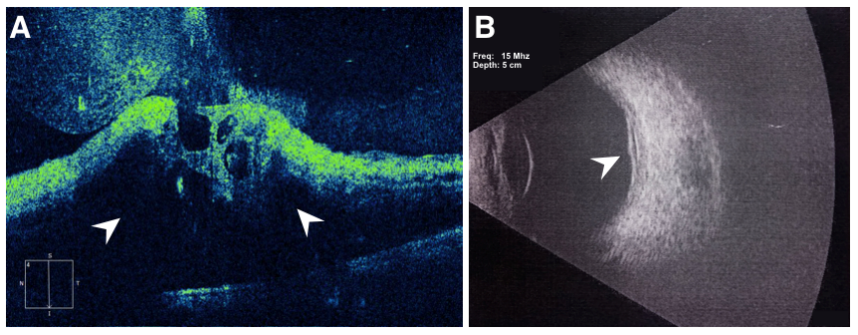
The optical coherence tomography image shows extensive macular edema seen as hyporeflective regions within the retina (arrowhead, 2A). Back shadowing due to superficial hemorrhages is also seen. Ultrasound imaging shows thickening of the retina over the posterior pole (arrowhead, 2B).
